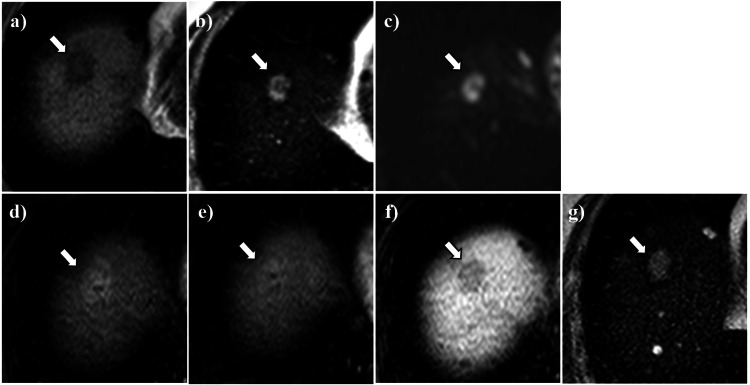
Abdominal magnetic resonance imaging. Abdominal magnetic resonance imaging (MRI) of the hepatic nodule (arrow). The hepatic nodule appeared hypointense on T1-weighted (a) image and hyperintense with hypointense area of its center on T2 (b) and diffusion-weighted (c) image. On gadolinium-ethoxybenzyl-diethylene-triamine-pentaacetic acid (Gd-EOB-DTPA)-enhanced arterial phase (d) the lesion showed hyperenhancement with hypointense area of its center that persists through equilibrium (e) phases. On Gd-EOB-DTPA-enhanced in the hepatobiliary phase showed hypointense (f). The follow-up MRI on the T2-weighted image after two months showed the hyperintense hepatic nodule slightly enlarged (g).
